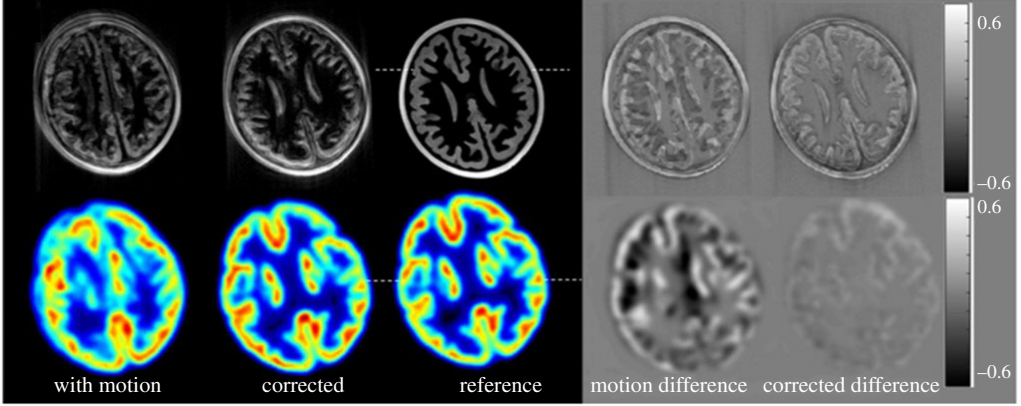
Motion correction results. A single slice of the motion-corrupted, corrected and reference PET and MRI images are shown. The difference images are presented in the fourth and fifth columns. The colour bars indicate the range of pixel intensities in the difference images (original images were scaled from 0 to 1). (Reproduced from Johnson *et al.* 2019. Rigid-body motion correction in hybrid PET/MRI using spherical navigator echoes. *Physics in Medicine and Biology*, 64, doi:10.1088/1361-6560/ab10b2 © Institute of Physics & Engineering in Medicine. Reproduced by permission of IOP Publishing. All rights reserved). [40]. (Online version in colour.)
